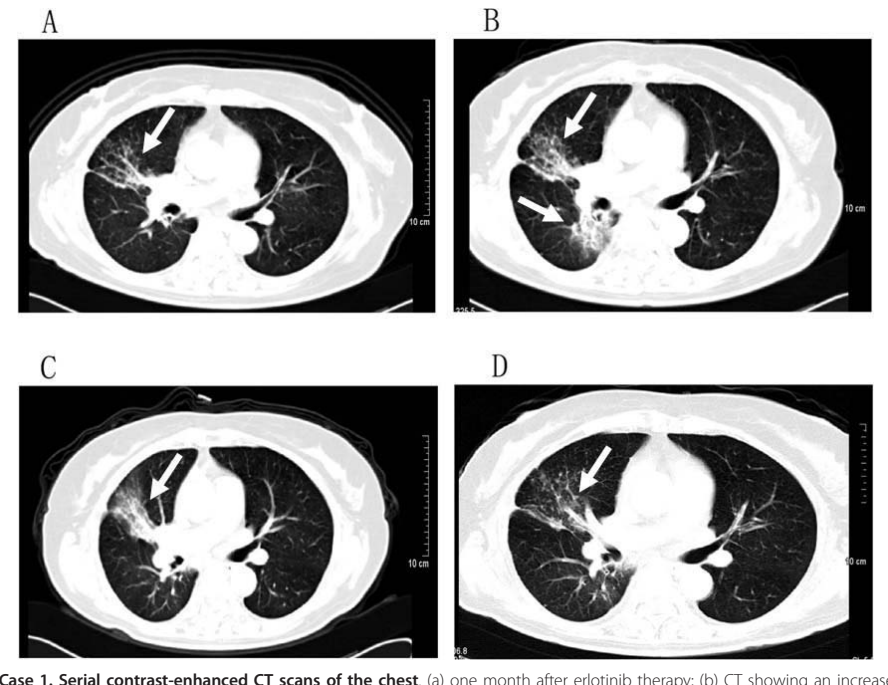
Case 1. Serial contrast-enhanced CT scans of the chest. (a) one month after erlotinib therapy; (b) CT showing an increase in size of the lung nodule after 12 months of erlotinib therapy; (c) CT showing a stable disease of the lung nodule after two cycles of chemotherapy; (d) CT showing a shrinkage of the tumor in the right lower lobe after 4 weeks of erlotinib re-challenge.
